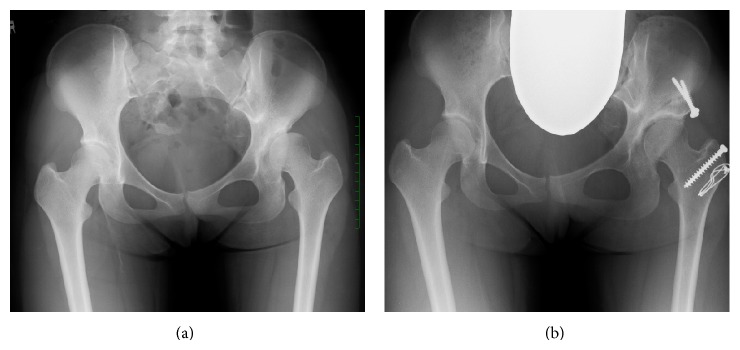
A 16-year-female with left hip subluxation associated with acetabular dysplasia and partial sacral agenesis treated with periacetabular osteotomy combined with allogeneic bone grafting. (a) Preoperative AP radiograph of the pelvis. (b) An AP radiograph obtained two years after the operation. The patient had achieved anatomical correction with an adequate bone stock in the hip joint.
